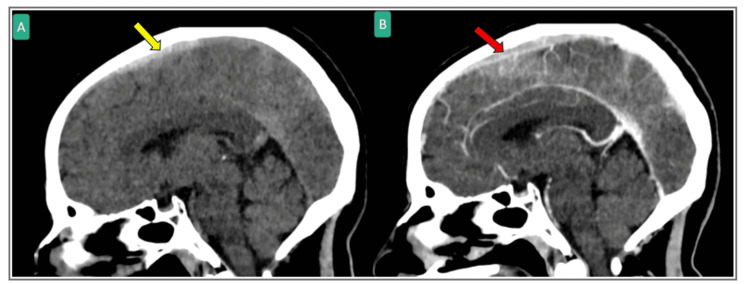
Sagittal head CT scan with non-contrast (A) and with contrast (B) showing hyperdensity at the superior sagittal sinus (Yellow arrow) that was confirmed by contrast to be superior sagittal sinus contrast filling defect (red arrow) suggesting cerebral venous thrombosis.
